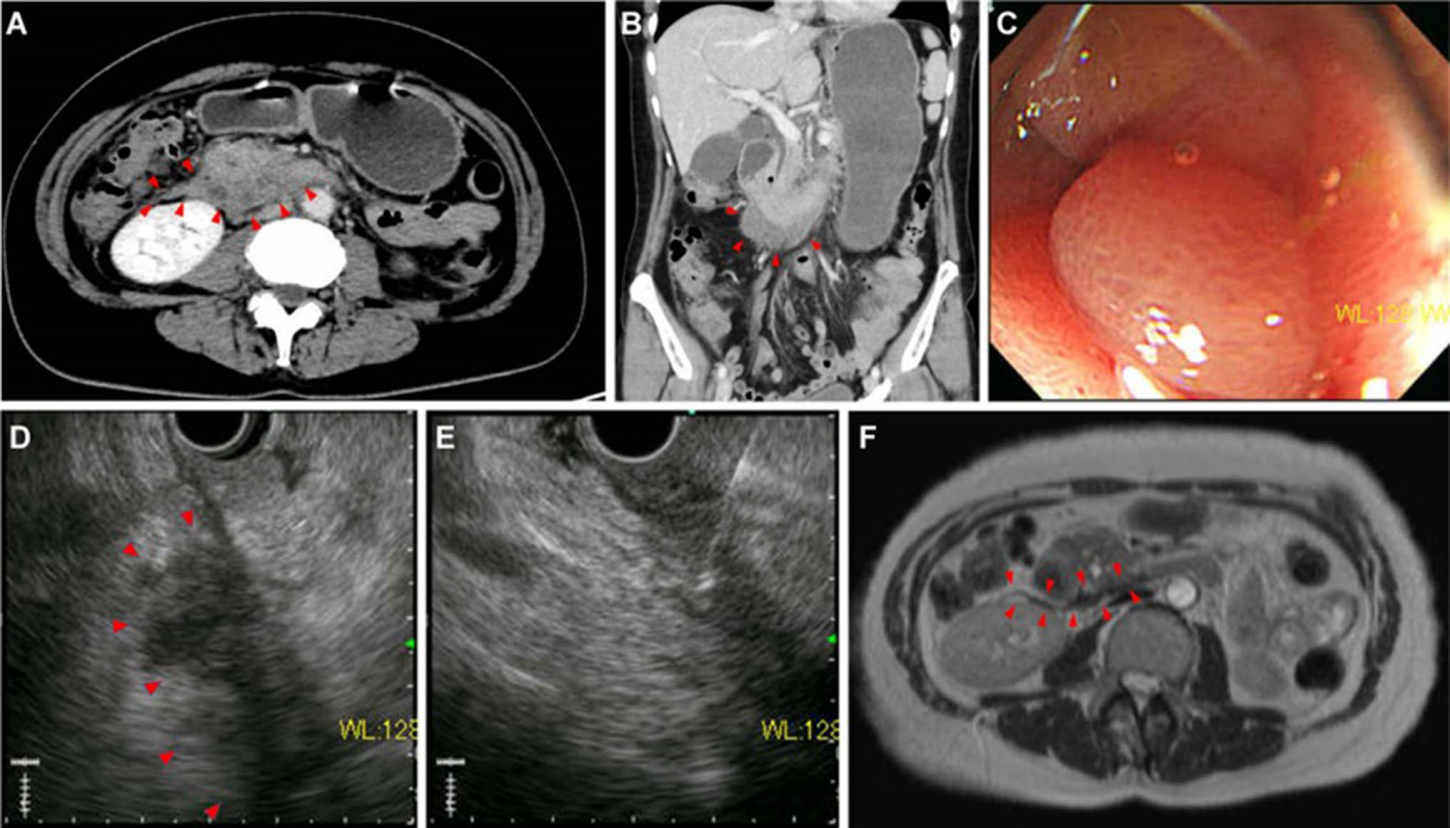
A 51-year-old female presenting with epigastric pain and vomiting (Case 1). a A low-density, band-shaped area spreading from the paraduodenal space to the right anterior pararenal extraperitoneal space was identified (arrowheads). The walls of the 2nd and 3rd part of the duodenum were thickened and in contact with the low-density area. b In coronal-reformatted images, the low-density area was situated on the caudal side of the 2nd to 3rd part of the duodenum and lead to its obstruction (arrowheads). c The esophagogastroduodenoscopy (EGD) on day 1 showed edematous, erythematous duodenal mucosa and narrowing of the lumen to the inferior duodenal angle. The endoscope did not pass through the lumen. d Endoscopic ultrasound (EUS) on day 5 revealed a band-shaped low echoic area just behind the 2nd part of the duodenum (arrowheads). e Transduodenal EUS-guided fine-needle aspiration/biopsy was performed to assess the possibility of a malignant lesion. f T2-weighted image of MRI on day 17 revealed no pancreatic tumor and no abnormal signal in the duodenal wall (arrowheads)
